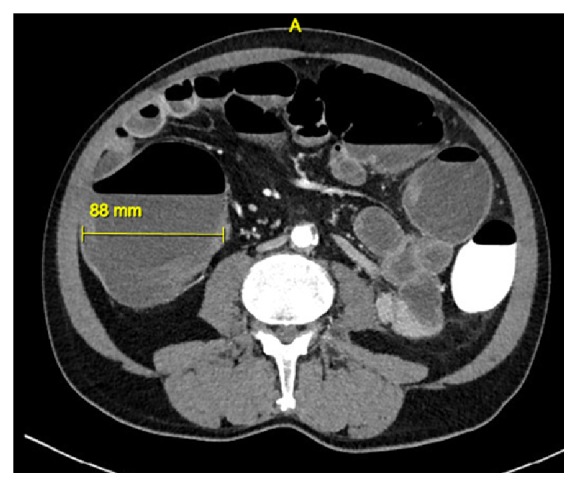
Abdominal CT scan of the abdomen after 300 mL rectal gastrografin and 100 mL intravenous nonionized iodine contrast. Status after partial sigmoidectomy. Gross colonic distension (Ø 88 mm), especially marked at the splenic flexure, without pathological bowel wall thickening. Distension of the terminal ileum, with signs of wall thickening. No free air or fluid, no suture leakage, no abscess, and no signs of ischemia.
